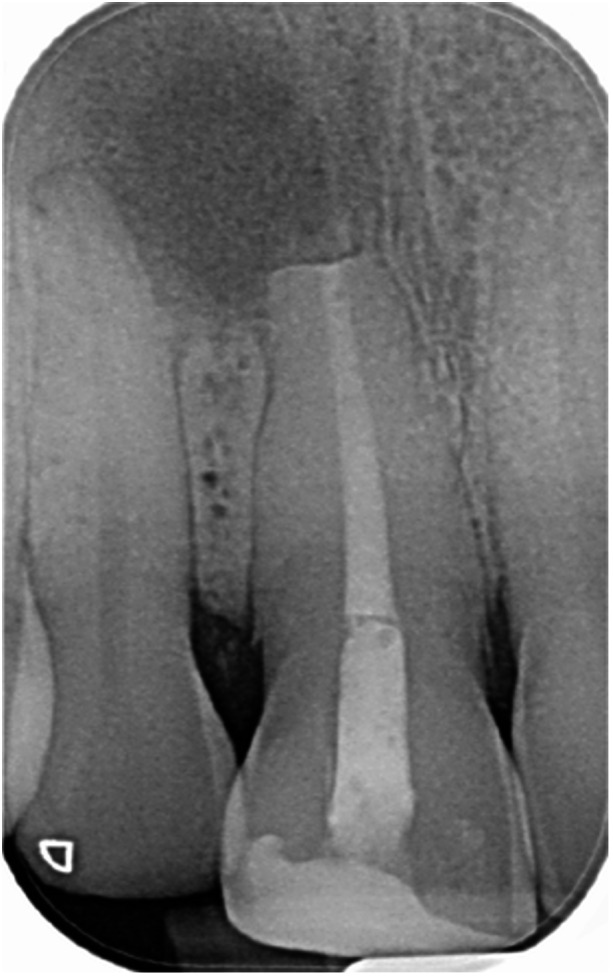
Case 13* (review was done at 4 months): The preoperative CBCT and periapical radiograph show an extensive periapical radiolucency associated with tooth 11, which has thinned the labial and palatal cortical plates. Apical surgery was performed on tooth 11 after the completion of a satisfactory nonsurgical root canal retreatment on the tooth. At 4 months, marked healing can be seen, evidenced by a reduction in the periapical radiolucency size associated with tooth 11.
